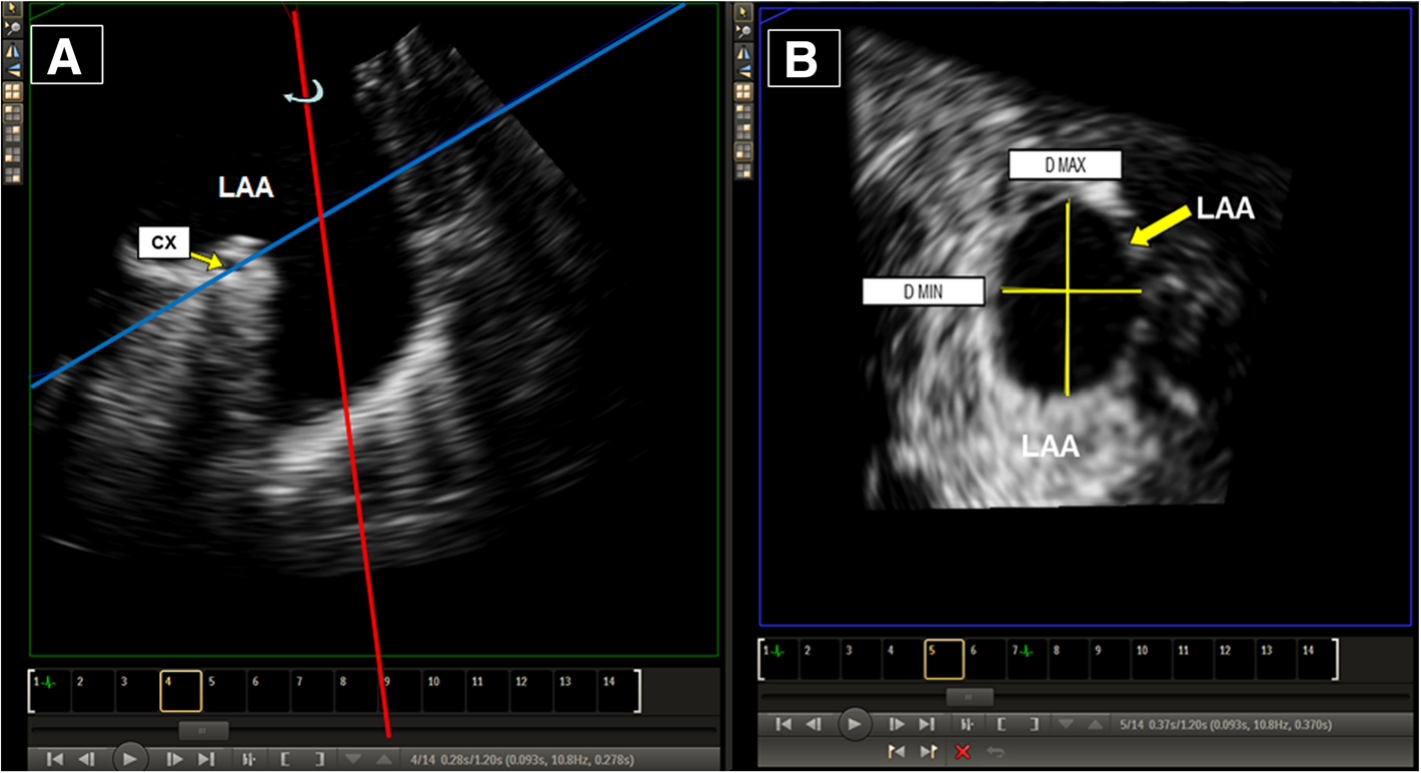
Three dimensional transesophageal echocardiography (3DTEE) measurements of left atrial appendage (LAA) using 360°, Rotational 3DTEE method (see text and panel a); and measurement of maximal (Dmax) and minimal (Dmin) diameters at the level of the circumflex (Cx) artery (panel b Video clip is attached)
